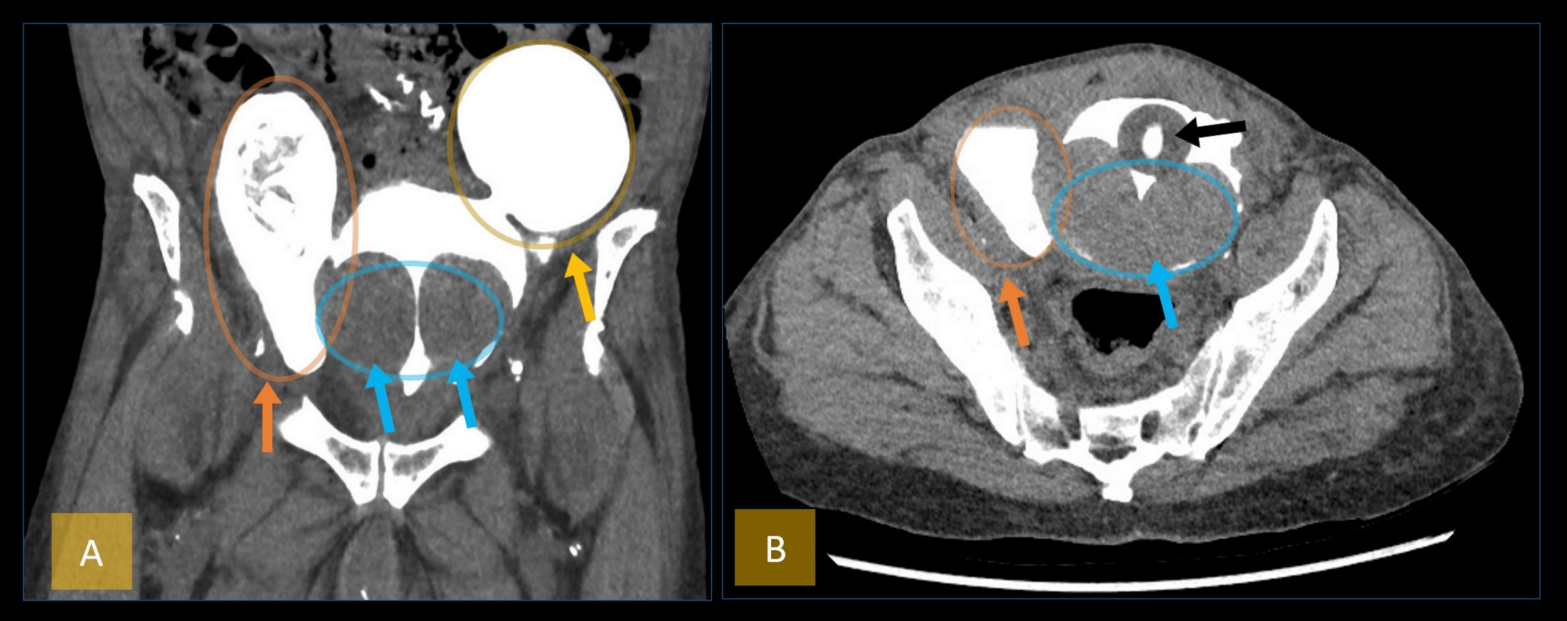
CT of the kidney, ureter, and bladder without contrast with retrograde cystogram demonstrating bilateral bladder diverticula and prostatic enlargement. (A) Coronal view: The orange arrow and circle highlight the right lateral bladder wall diverticulum. The yellow arrow and circle indicate the left lateral bladder wall diverticulum. The blue arrow and circle show the markedly enlarged median prostatic lobe protruding into the bladder. (B) Axial view: The orange arrow and circle mark the right lateral diverticulum. The Foley catheter balloon is identified by the black arrow. The blue arrow and circle point to the markedly enlarged median prostatic lobe protruding into the bladder, consistent with bladder outlet obstruction.
